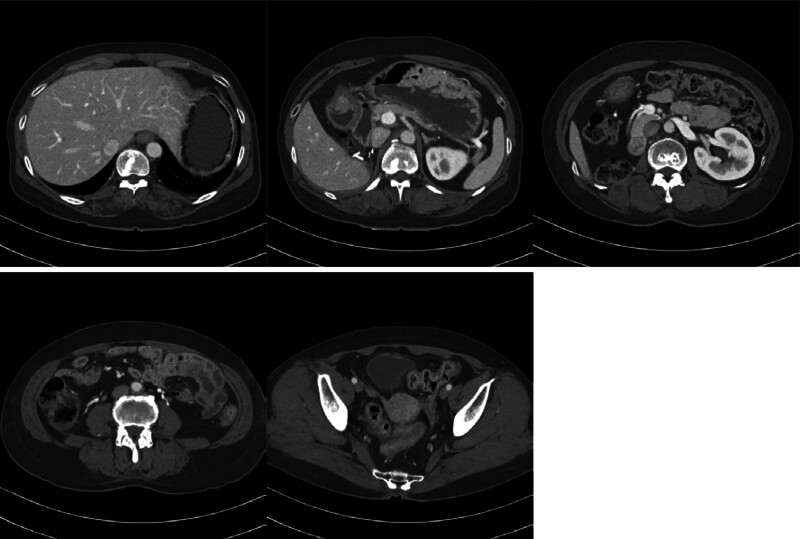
Abdominopelvic CT performed 3 months after discharge.
